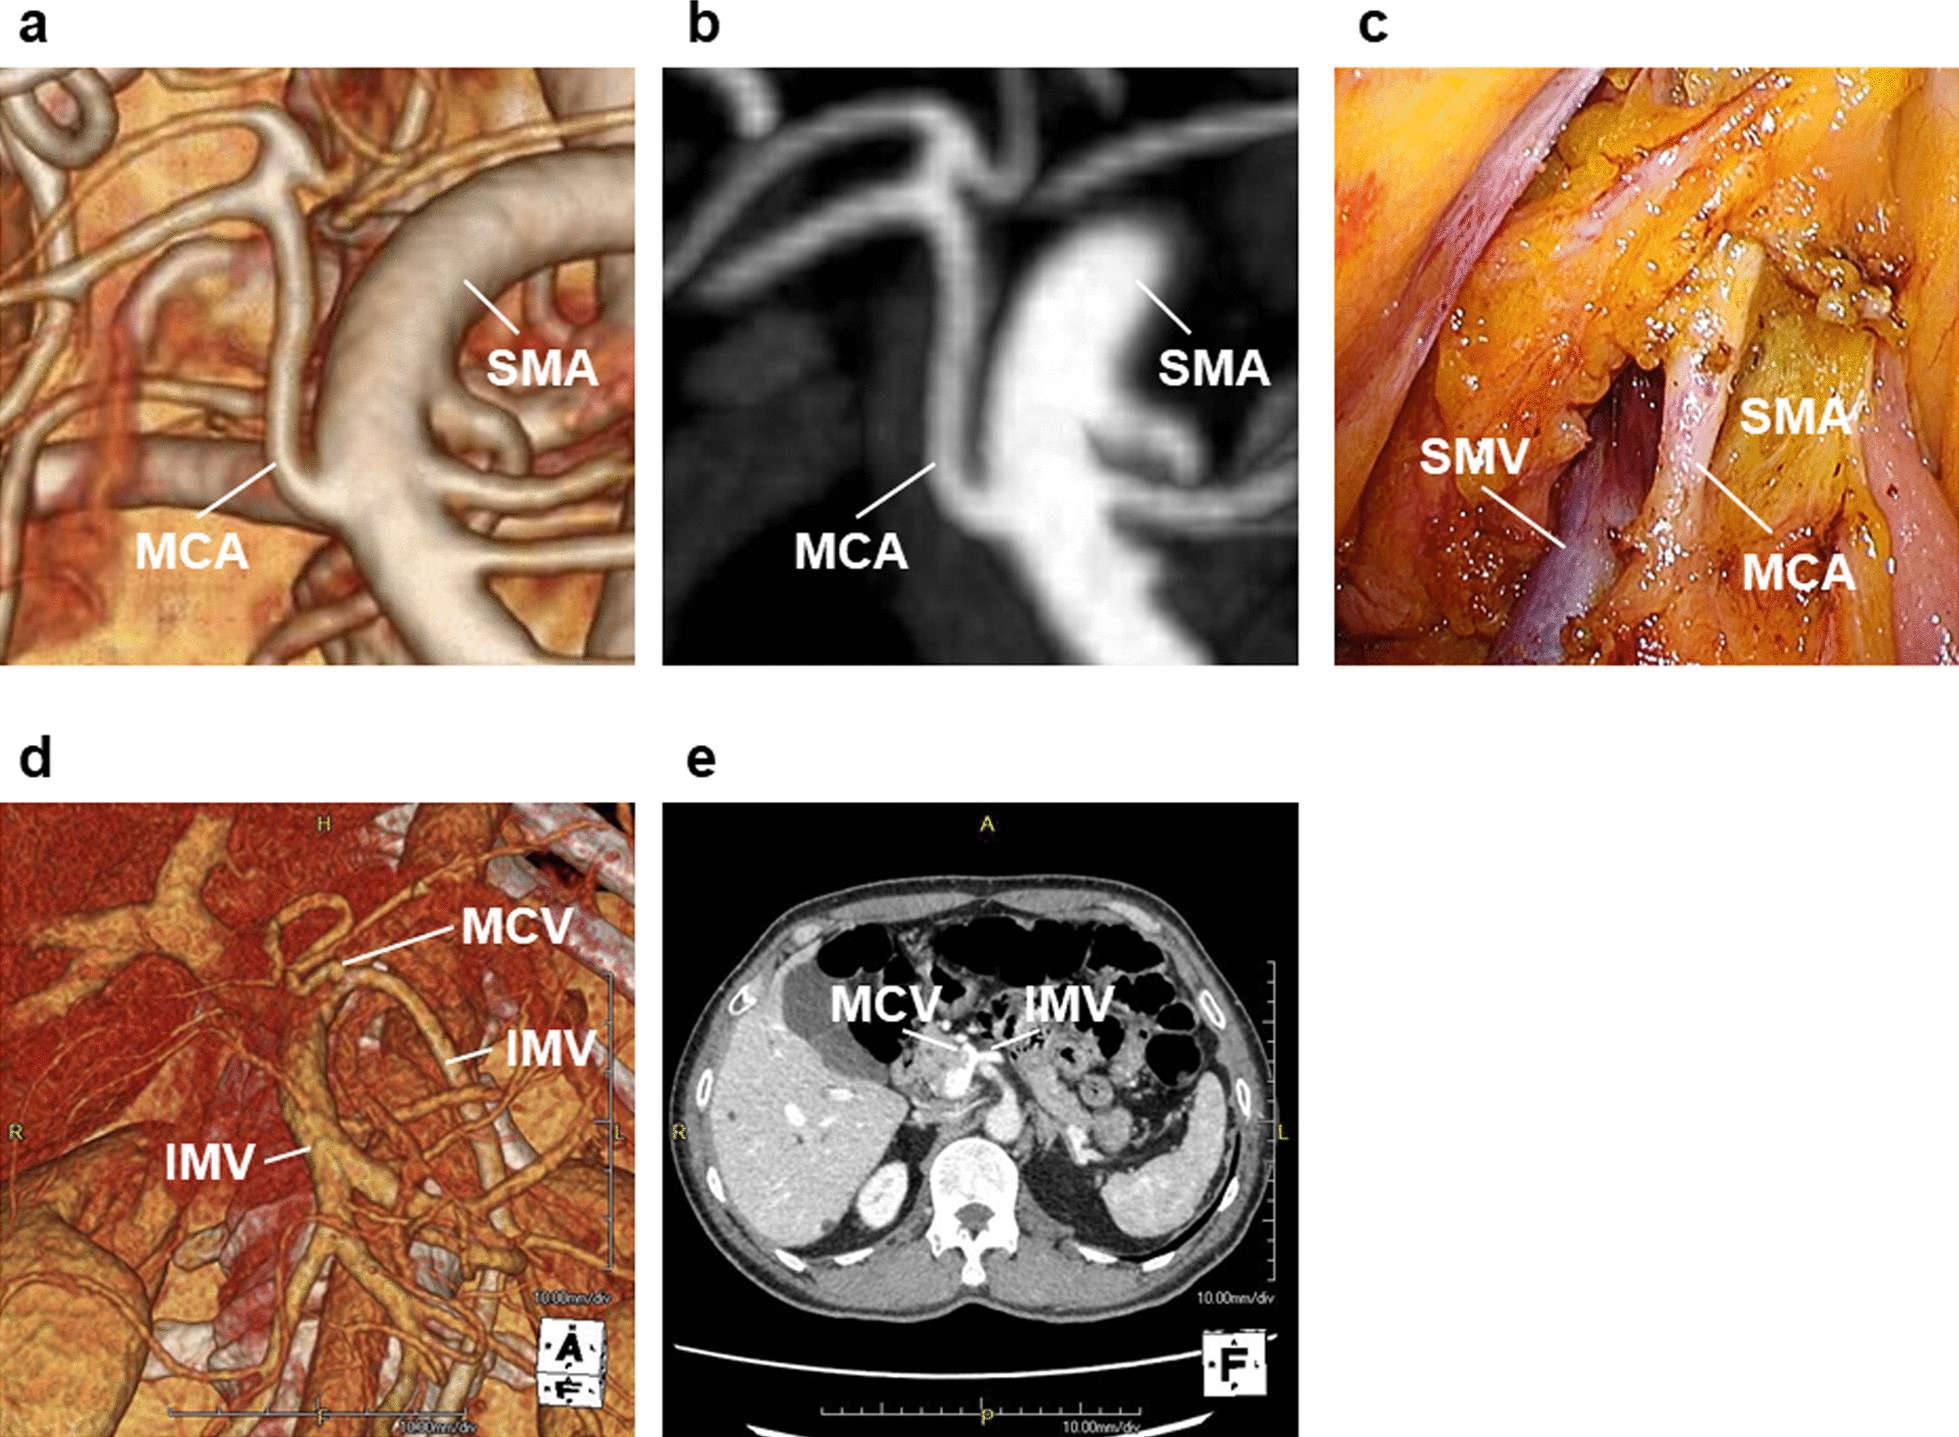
CT and operation findings. a 3D CT of MCA Type A. b MIP (Maximum Intensity Projection) CT of MCA Type. c The operation field of MCA Type A. d 3D CT of MCV Type C. There was no MCV on the right side of SMV. e CT of MCV Type C
